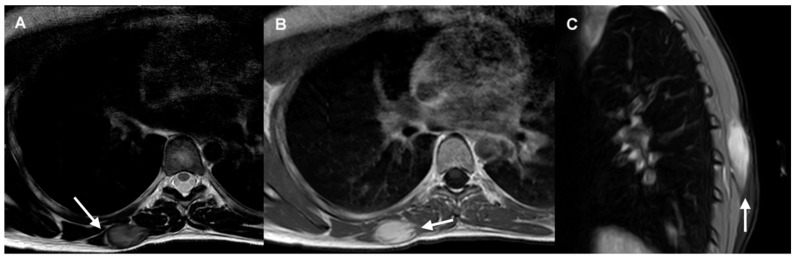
DT of the right distal trapezius muscle (Scale 11.9×). (A) The axial T2-weighted MRI sequence demonstrates a well-defined mass with intermediate signal and hypointense internal collagen bundles. The “split-fat sign” is shown in its lateral margin (arrow). (B) Axial post-contrast T1-weighted MRI sequence shows homogeneous mass enhancement and “staghorn sign” (arrow). (C) Sagittal fat-suppressed image shows “fascial tail sign” (arrow).
